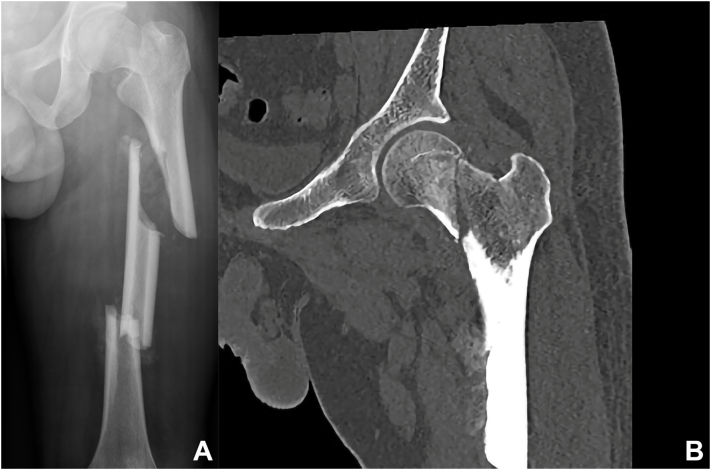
Preoperative imaging. A: X-ray showed ipsilateral left femoral neck and shaft fracture with obvious displacement. B: CT scan showed the fracture line of the left femoral neck is from subcapital to basicervical, and the Pauwels angle was more than 50 degrees.
